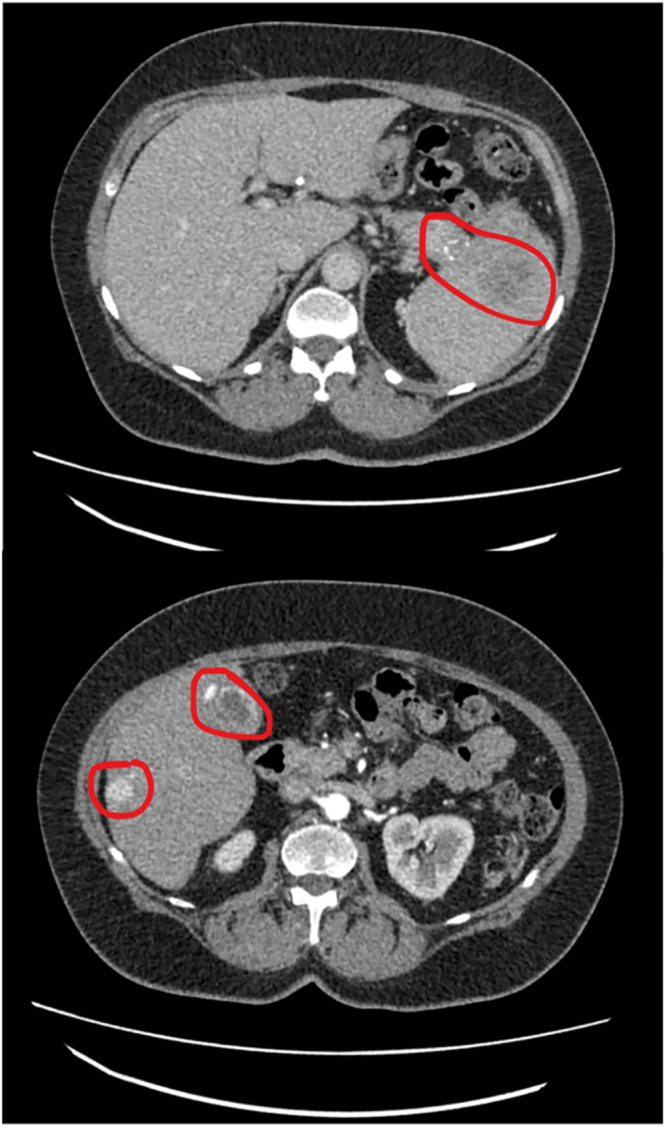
Case 1: contrast-enhanced axial CT images of the abdomen showing calcified pancreatic tail mass with extension into the spleen, along with multiple liver metastases.
